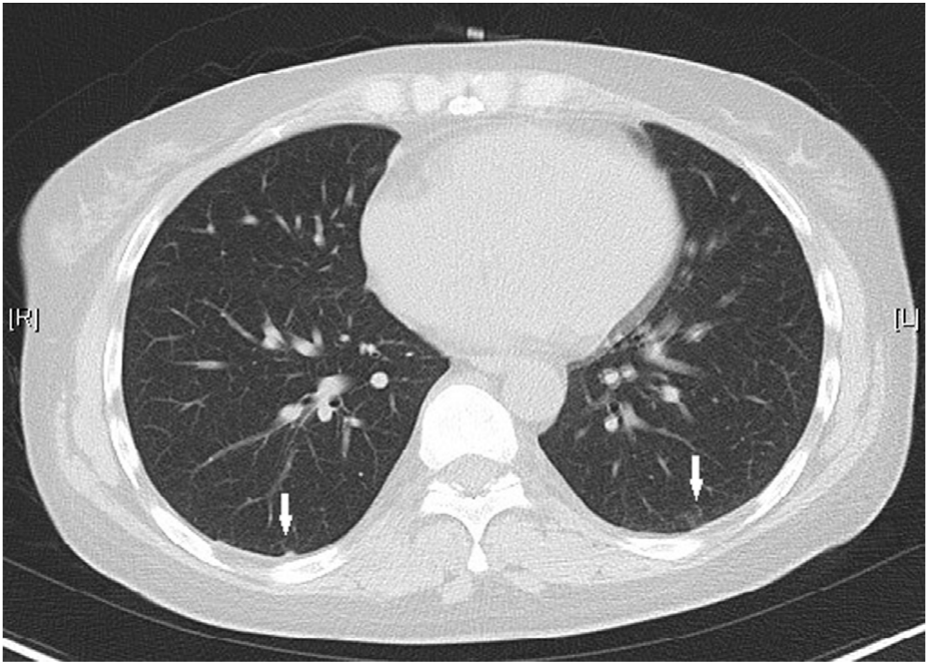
Chest CT showed small flaky high-density foci near the pleura on both sides (white arrows).
